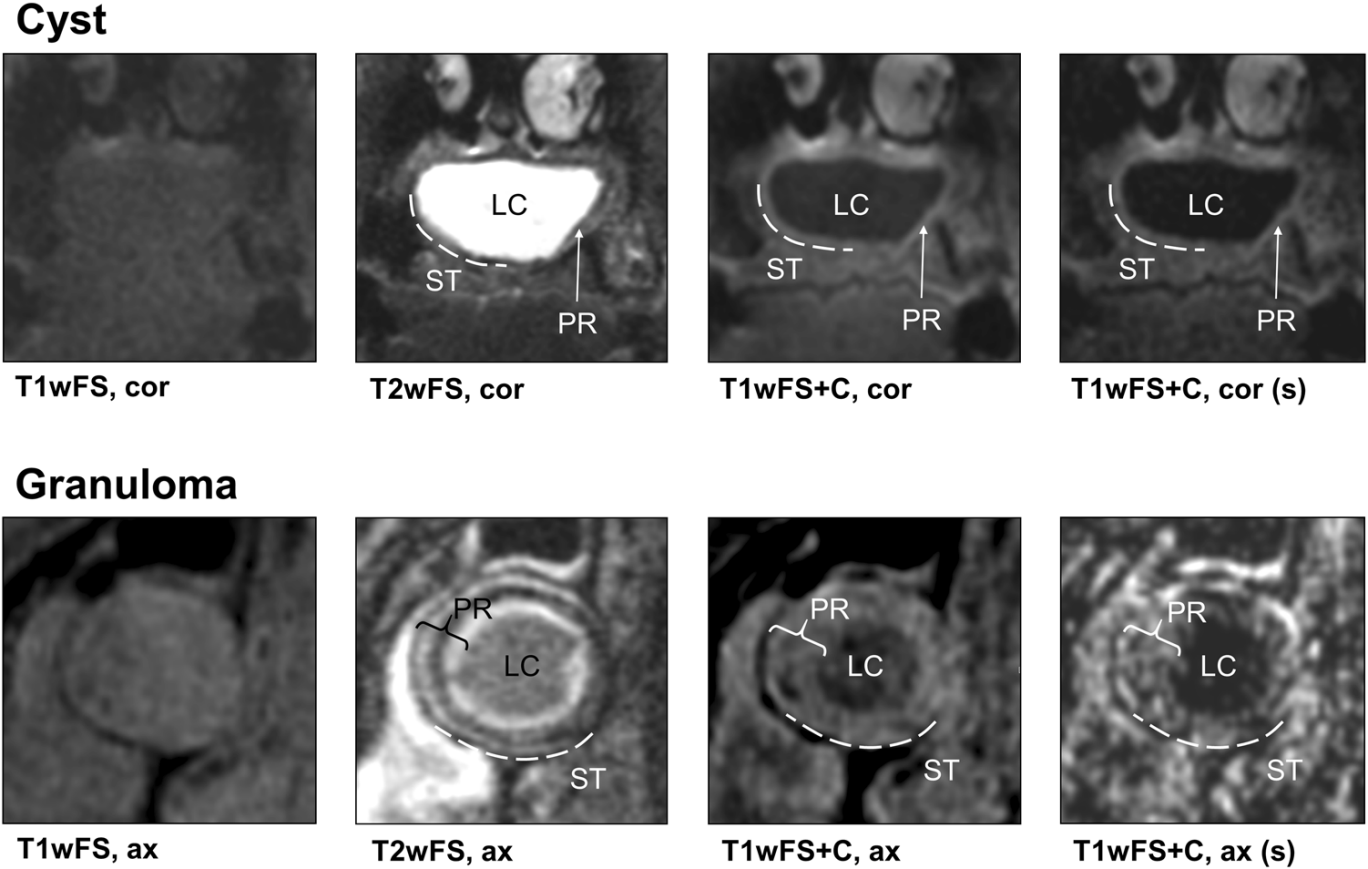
Typical MRI features of a periapical cyst and a granuloma. Unlike T2wFS and T1wFS+C, non-contrast-enhanced T1w/T1wFS images did not enable a reliable delineation of the peripheral rim. Six features allowed for differentiating between cysts and granulomas: (1) outer margin of the lesion (cysts: well-defined, granulomas: ill-defined). (2) Texture of the PR in T1wFS+C (cysts: homogeneous, granulomas: inhomogeneous). (3) Texture of the LC in T2wFS (cysts: homogeneous, granulomas: inhomogeneous). (4) Involvement of the ST in T2wFS (cysts: no, granulomas: yes). (5) Involvement of the ST in T1wFS+C (cysts: no, granulomas: yes) and (6) Maximum thickness of the PR (cysts: thin, granulomas: thick). T1wFS T1-weighted with fat saturation, T2wFS T2-weighted with fat saturation, T1wFS+C T1-weighted with fat saturation+contrast agent, (s) subtraction, cor coronal, ax axial, PR peripheral rim, LC lesion center, ST surrounding tissue
